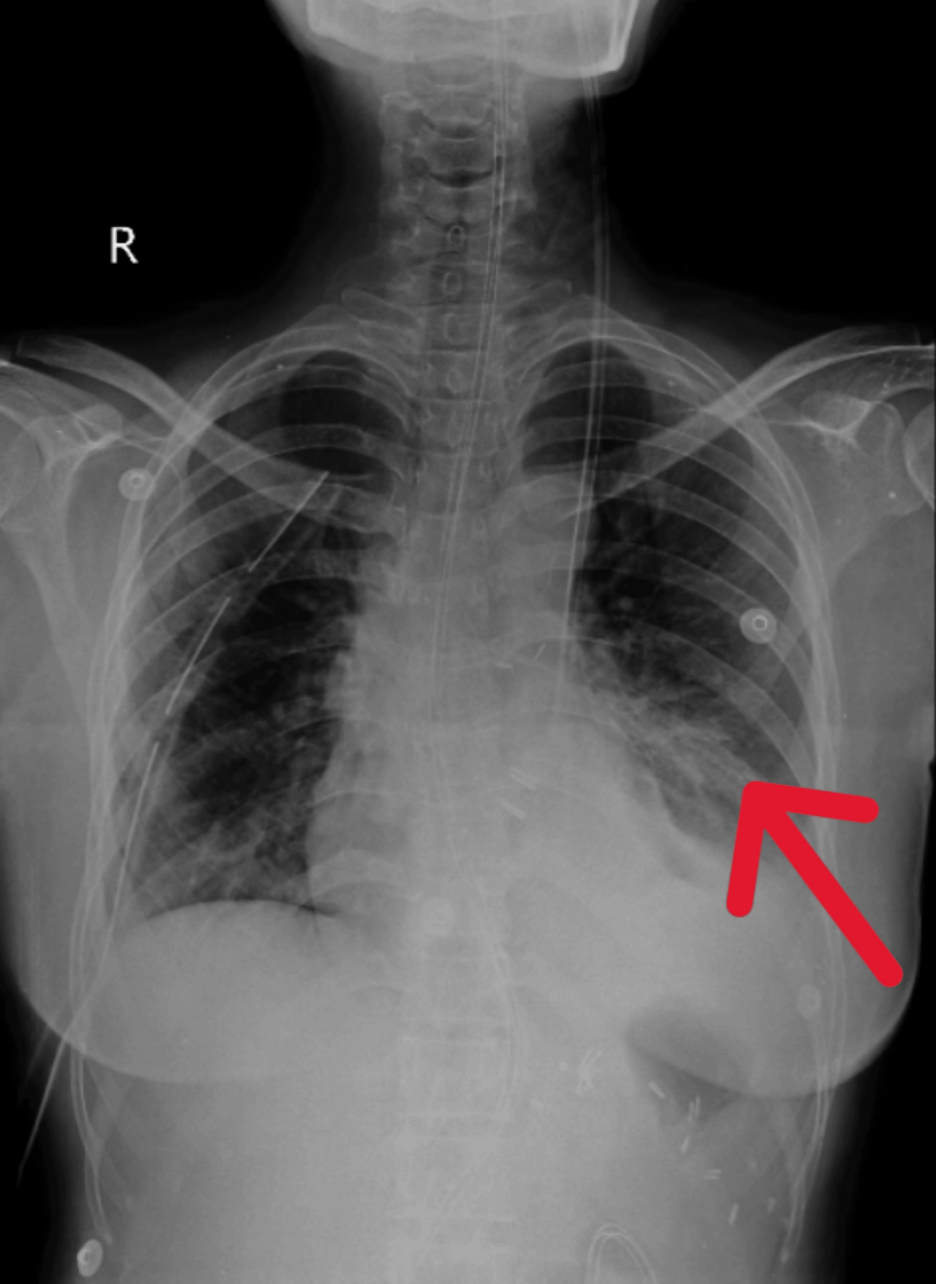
X-ray AP view of post-rehabilitation The red arrow shows the improved lung field. AP: anteroposterior view
